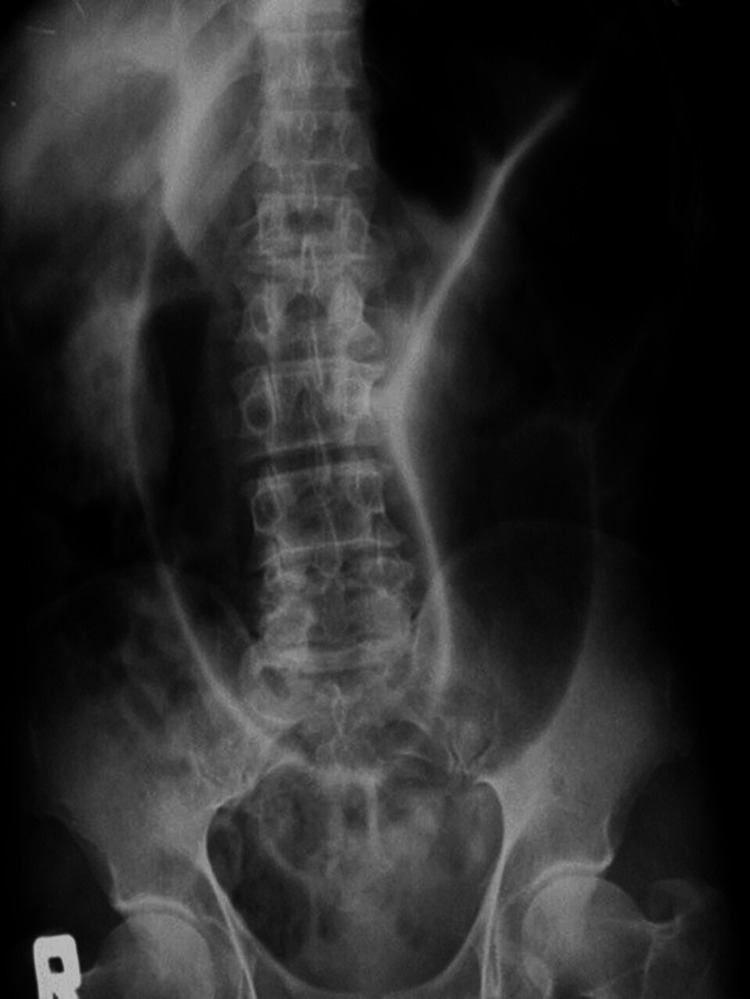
"Coffee Bean" sign on erect abdominal radiograph.
